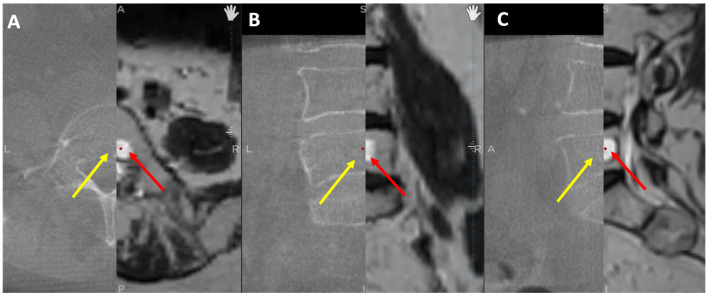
O-arm and MRI fused images: (A) Axial view; (B) Coronal view; (C) Sagittal view. The left half of each view displays the O-arm scan. The right half displays the MRI scan. Red arrows indicate the tumors and yellow arrows show where the tumor should be visible.
